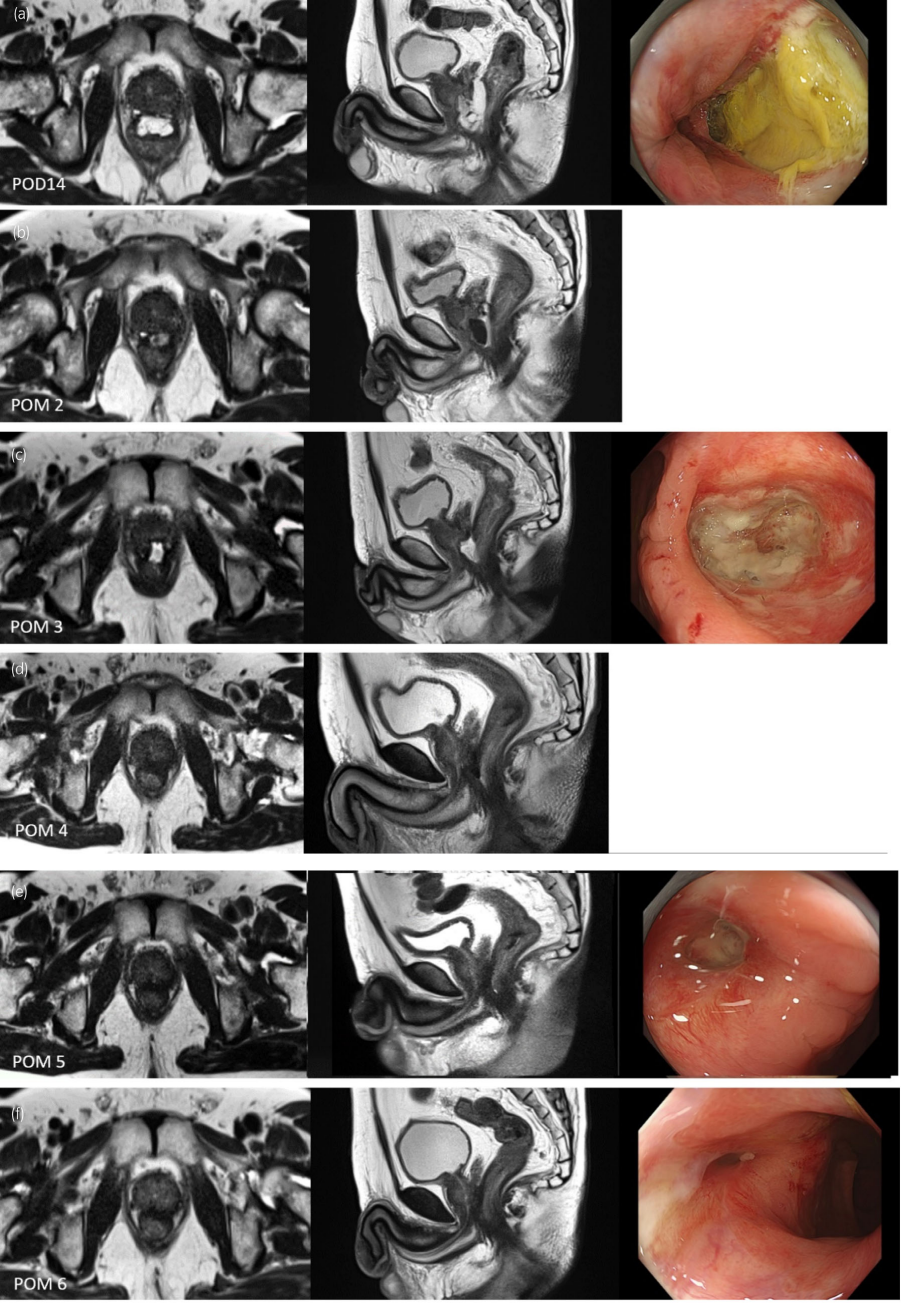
Temporal changes in T2‐MRI and CS findings.
